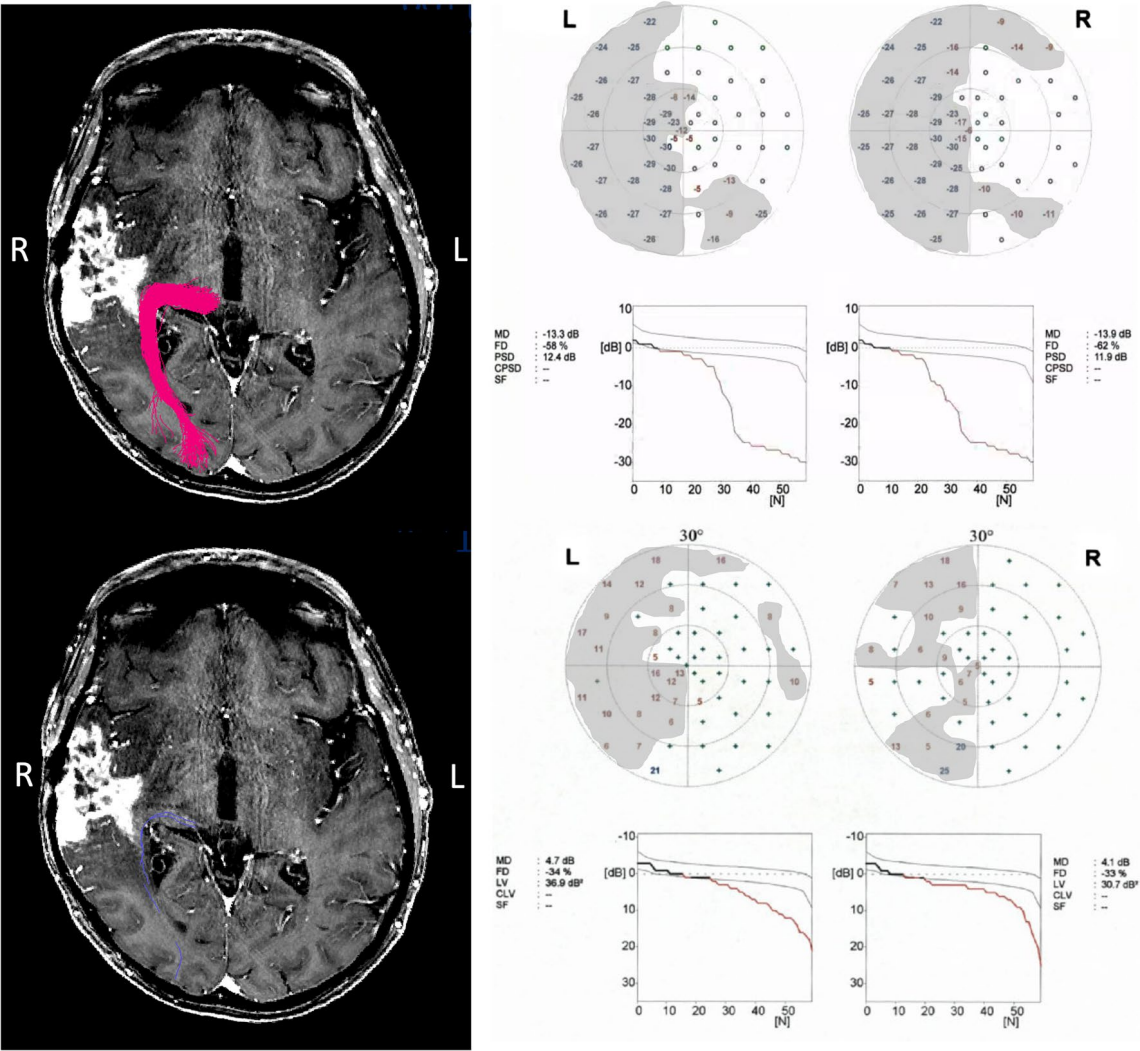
Case report for patient with improvement of visual field deficits and lesion in the medial temporal lobe, intrinsic to the OR based on QBI-FT, lateral according to DTI-FT. Left column, upper line: QBI-FT. Left column, lower line: DTI-FT. Right column, upper line: preoperative perimetry. Right column, lower line: postoperative perimetry. VFDs shaded in gray with defect depth < −5dB. MRI = magnetic resonance imaging; FT = fiber tractography; QBI = q-ball imaging; DTI = diffusion tensor imaging
